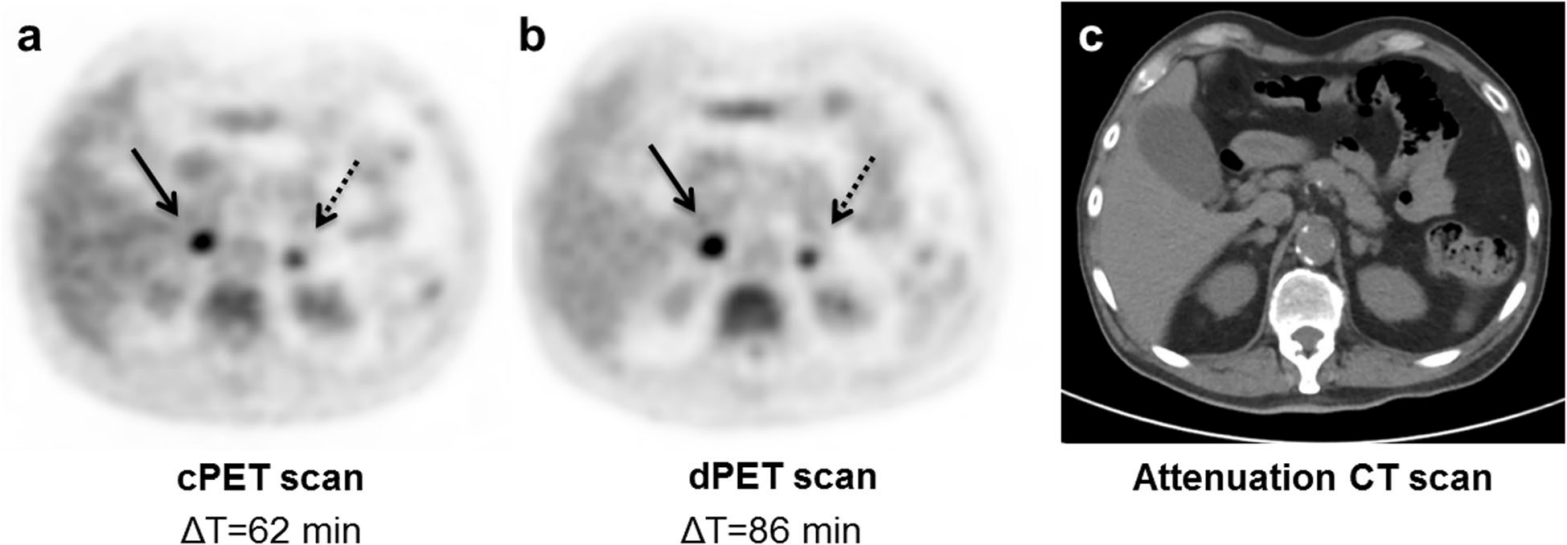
Axial FDG-PET/CT images (a, b and c) from a lung cancer patient with bilateral adrenal gland metastases showing higher SUVs on the dPET scan (b) that was acquired 24 min after the cPET scan (a). The left-gland metastasis (diameter 11 mm) showed ΔSUVs of 7% (SUV_mean_), 10% (SUV_peak_) and 15% (SUV_max_). ΔSUVs of the right-gland metastasis (diameter 14 mm) were 13% (SUV_mean_) and 11% (SUV_peak_ and SUV_max_). In this case the impact of the digital scanner cannot be separated from the SUV rise caused by the prolonged FDG-uptake. Meanwhile, the visual image quality of both PET scans appeared comparable in terms of image noise, texture and FDG-uptake as intended with an EARL-compatible protocol
